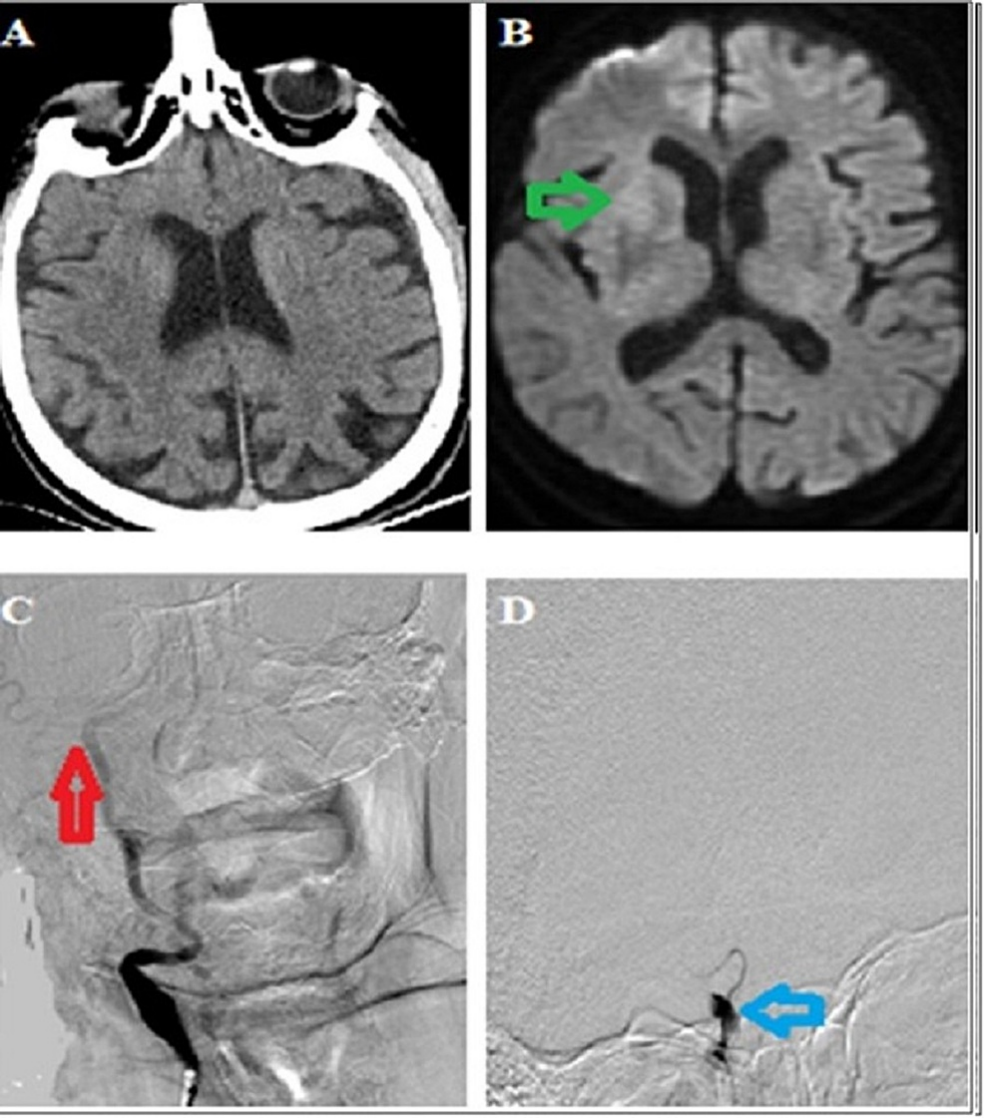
(A) The Alberta stroke program early CT score was 10 on non-contrast cranial CT 2.5 hours after symptom onset. (B) Hyperintensity at the level of the right basal ganglia in diffusion-weighted imaging suggesting a hyperacute infarction (green arrow). (C) and (D) Slow flow and carotid T occlusion in right internal carotid injection (red and blue arrow).
